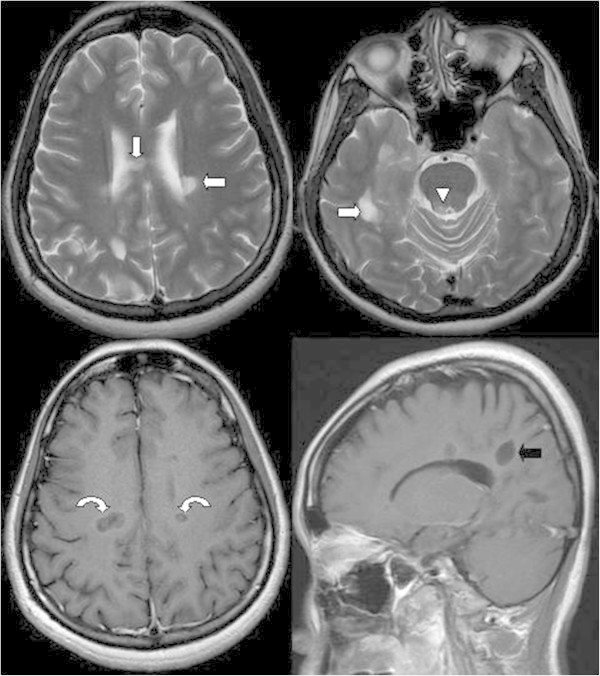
Hyperintense lesions in the bilateral periventricular white matter (arrow), body of the corpus callosum (arrow), and periaqueductal grey matter (arrowhead) are noted on the T2-weighted image. After gadolinium contrast injection, ring enhancement over these lesions is noted on the T1-weighted image (curved arrows), which is suggestive of active demyelination. In the sagittal view, multiple sclerosis plaques with a typical perpendicular orientation at the callososeptal interface are noted (Dawson’s fingers) (black arrow).
